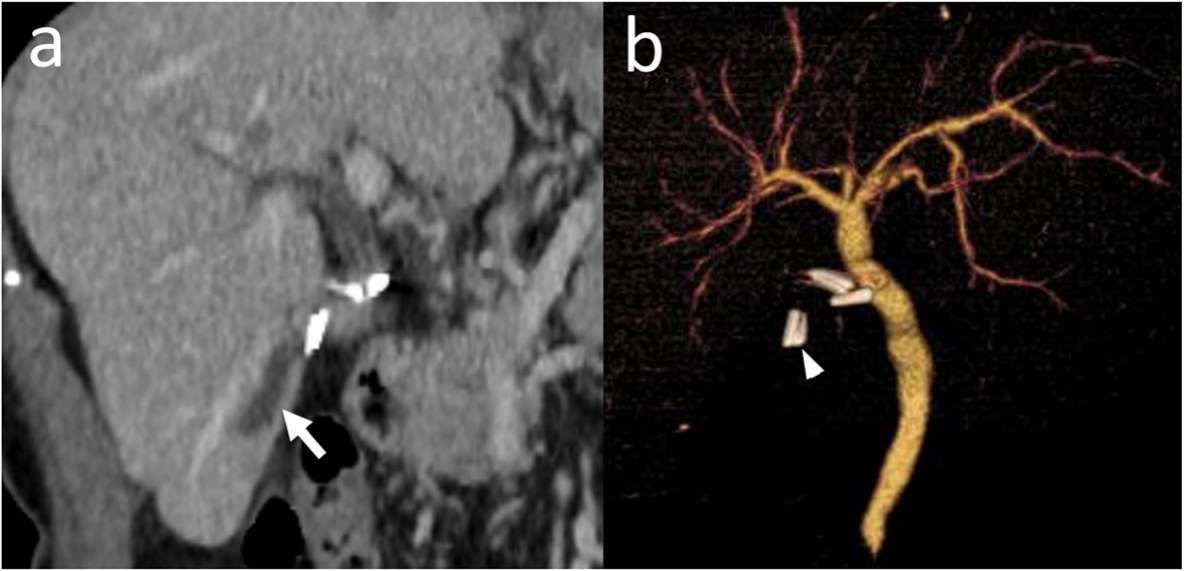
a The computed tomography scan performed 6 months after the surgery reveals the dilatation of the ventral branch of right posterior inferior segmental bile duct (arrow). b Drip infusion cholecystocholangiography-computed tomography performed on postoperative day 11 reveals the clip on the cholecystohepatic duct (arrowhead) just below the cystic duct clips
